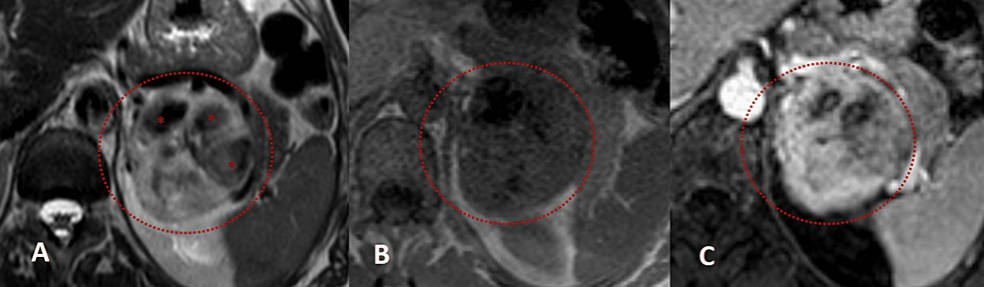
Abdominal magnetic resonance imaging: axial T2-weighted sequence (A), axial in-phase T1-weighted sequence (B), and axial dynamic sequence, portal venous phase (C). Left adrenal solid mass (circles), measuring 7 cm × 6.5 cm, with diffuse high and heterogeneous signal on T2, with internal areas (*) of low signal (A), with diffuse and heterogeneous hyposignal on T1 in-phase sequence (B). After administration of paramagnetic contrast, the lesions showed intense and heterogeneous enhancement (C).
